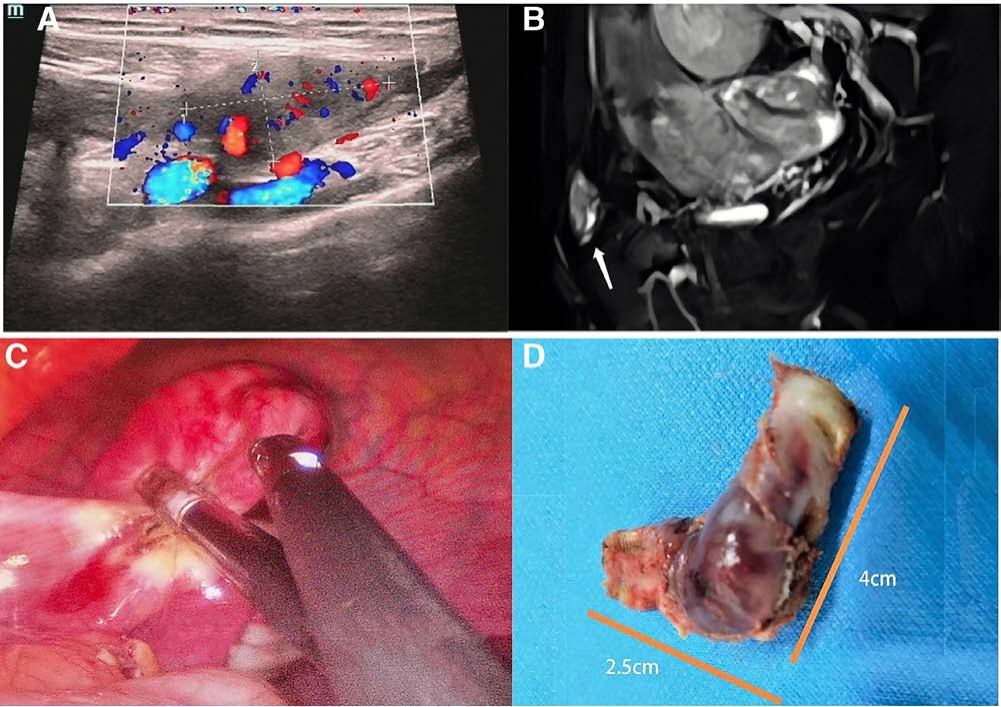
The imageology of case 1. (A) Sonography revealed a 2cm*2cm mass in the left groin area. Sagittal T2W MRI showed a mass in the left inguinal canal (white arrow). (C) Rudimentary uterus herniated through the internal inguinal ring. (D) The removed uterus. MRI = magnetic resonance imaging.
